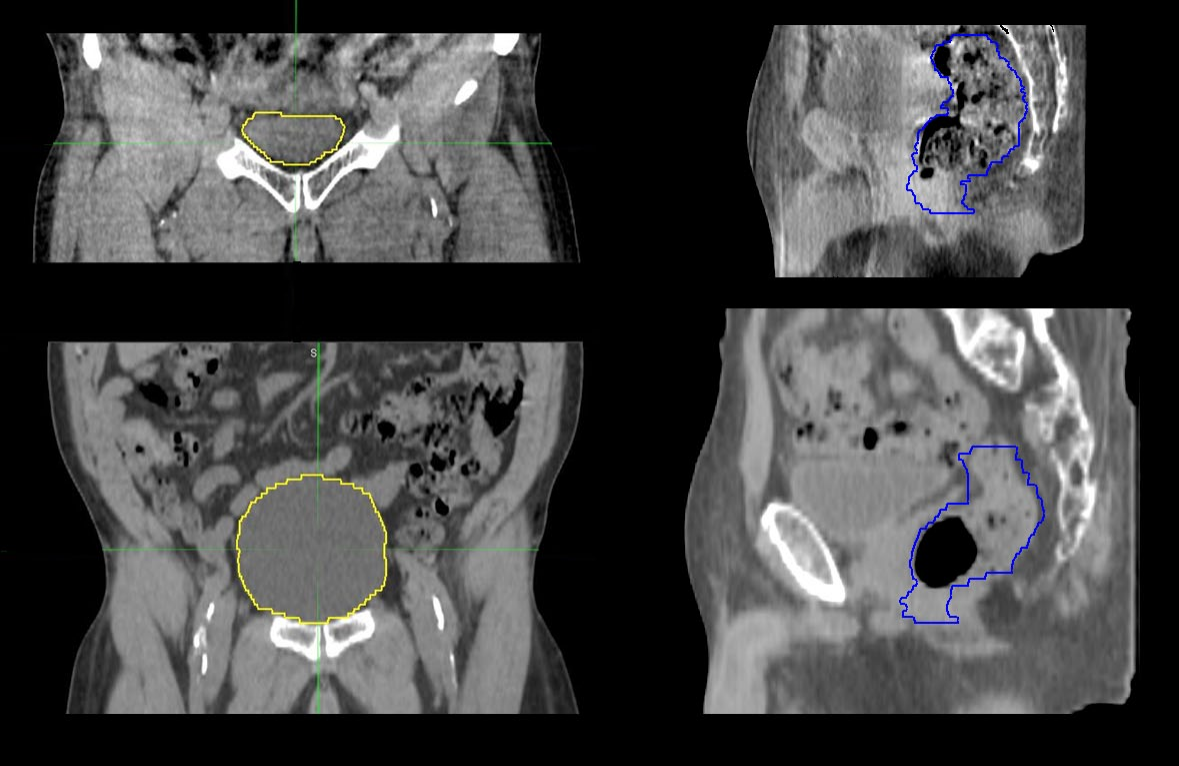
Change in bladder (left) and rectum (right) volume on P‐CT (bottom) and daily CBCT.
